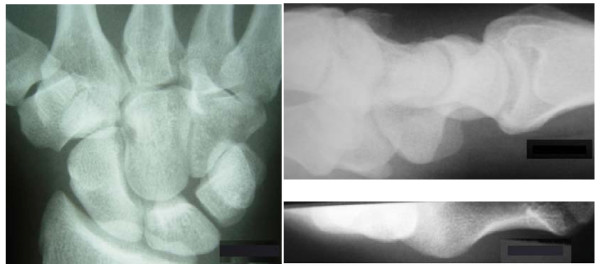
Anteroposterior, lateral and carpal tunnel x-ray views (clockwise from left).
